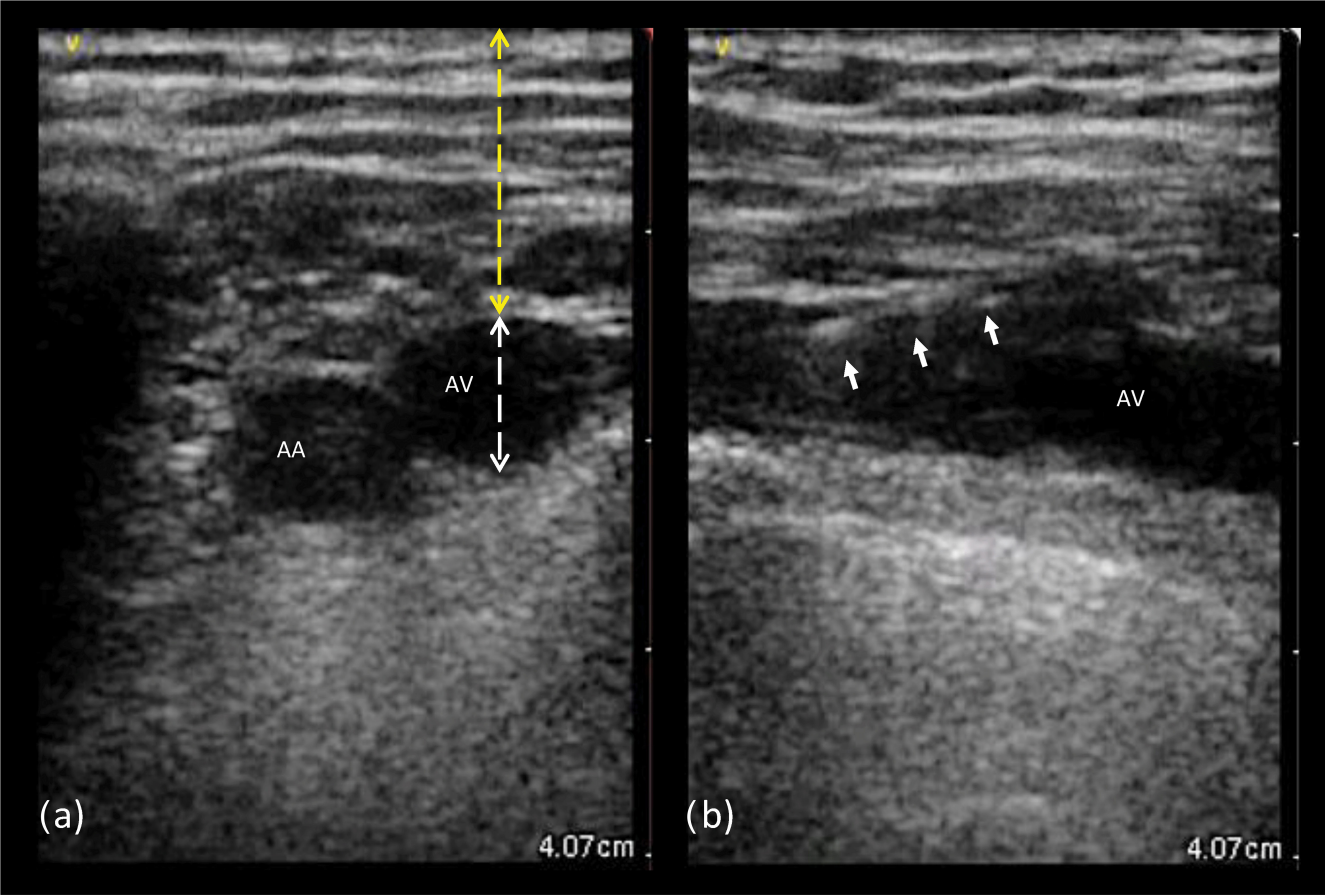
Ultrasound images from left axillary vein. (a) Transverse view:
